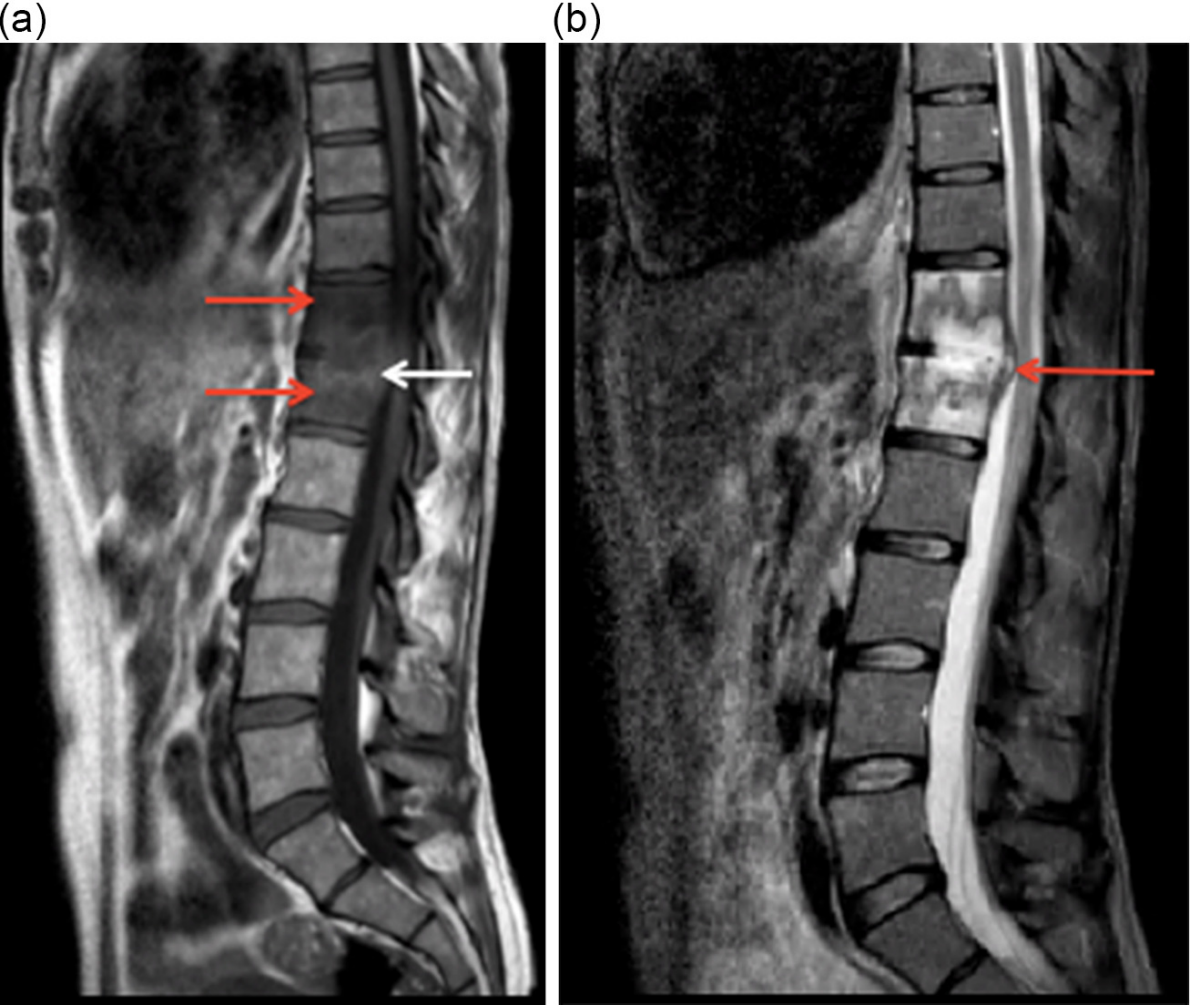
(a) T1-weighted sagittal MRI scan. Red arrows indicate areas of marrow oedema. The white arrow indicates a hyperintense rim (penumbra sign) surrounding the fluid signal intensity within the T12–L1 disc space. (b) STIR (short T1 inversion recover) sagittal MRI scan. The red arrow indicates the extension of disc space fluid.
